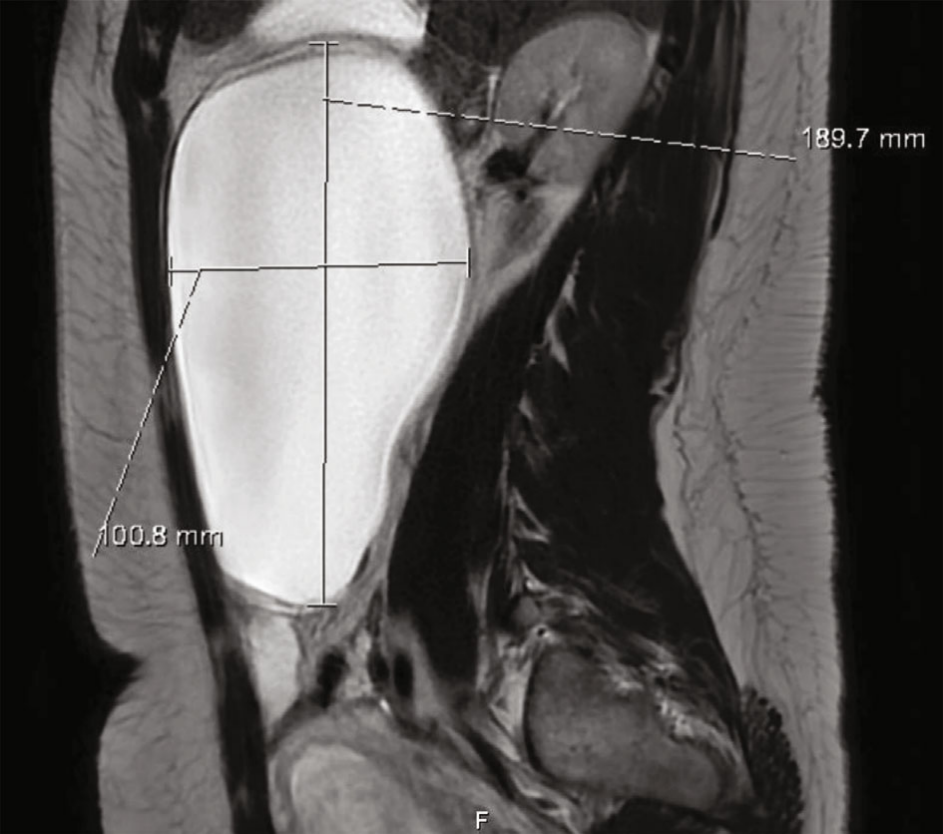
Sagittal view (MRI) of the right adnexal cyst. Note its simple appearance.
